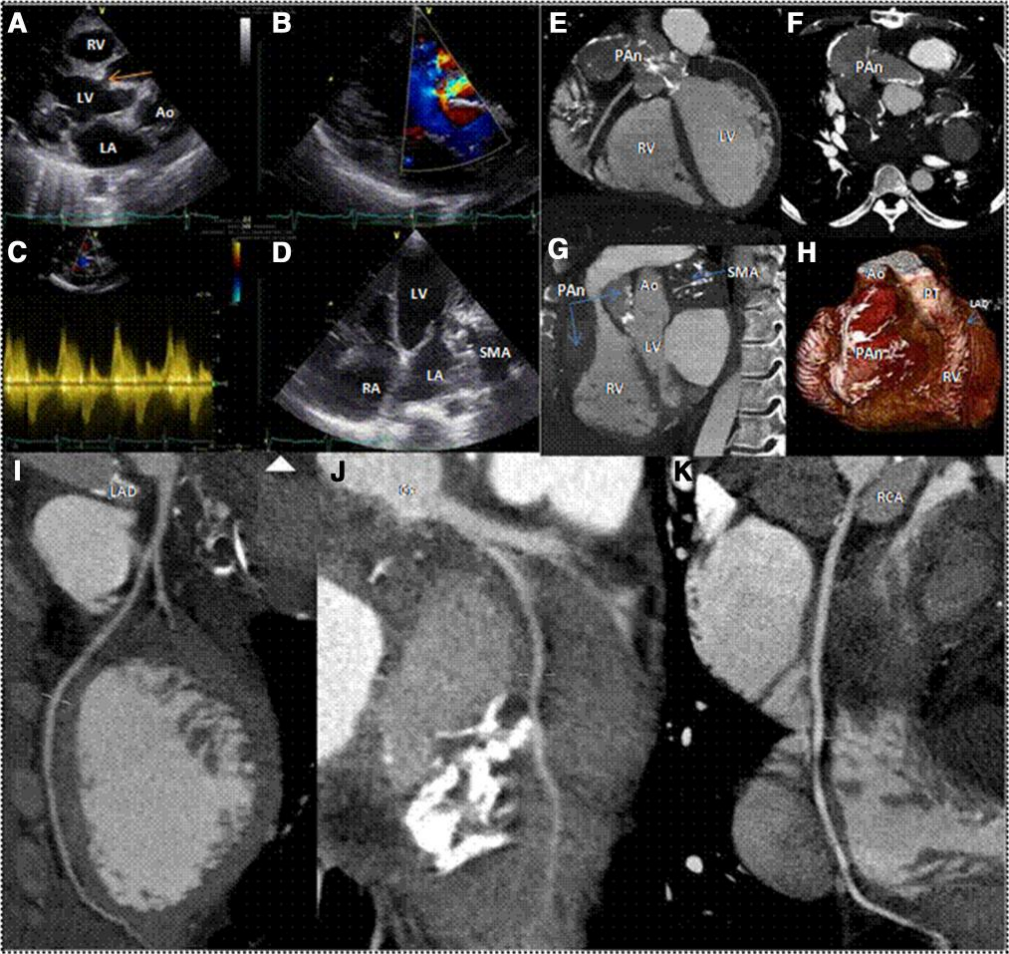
A 36-year-old male patient presented with dyspnoea, fatigue, and pre-syncope episodes during moderate exertion. Relevant history included surgery for a subvalvular mitral aneurysm (SMA) performed 14 years earlier. Although surgery was done suspecting a type A aortic aneurysm, the diagnosis was not confirmed intraoperatively. Detailed surgical notes were not available. Physical examination revealed a systolic-bar murmur in the third left intercostal space. 2D-transthoracic echocardiography and Doppler study displayed a large structure linked to the left ventricle through a narrow neck (*Panel A*, arrow), showing a turbulent jet filling a pseudoaneurysm (*Panel B*) with a high gradient between the ventricle and the pseudoaneurysm (*Panel C*). An operated SMA aneurysm was also observed (*Panel D*).
